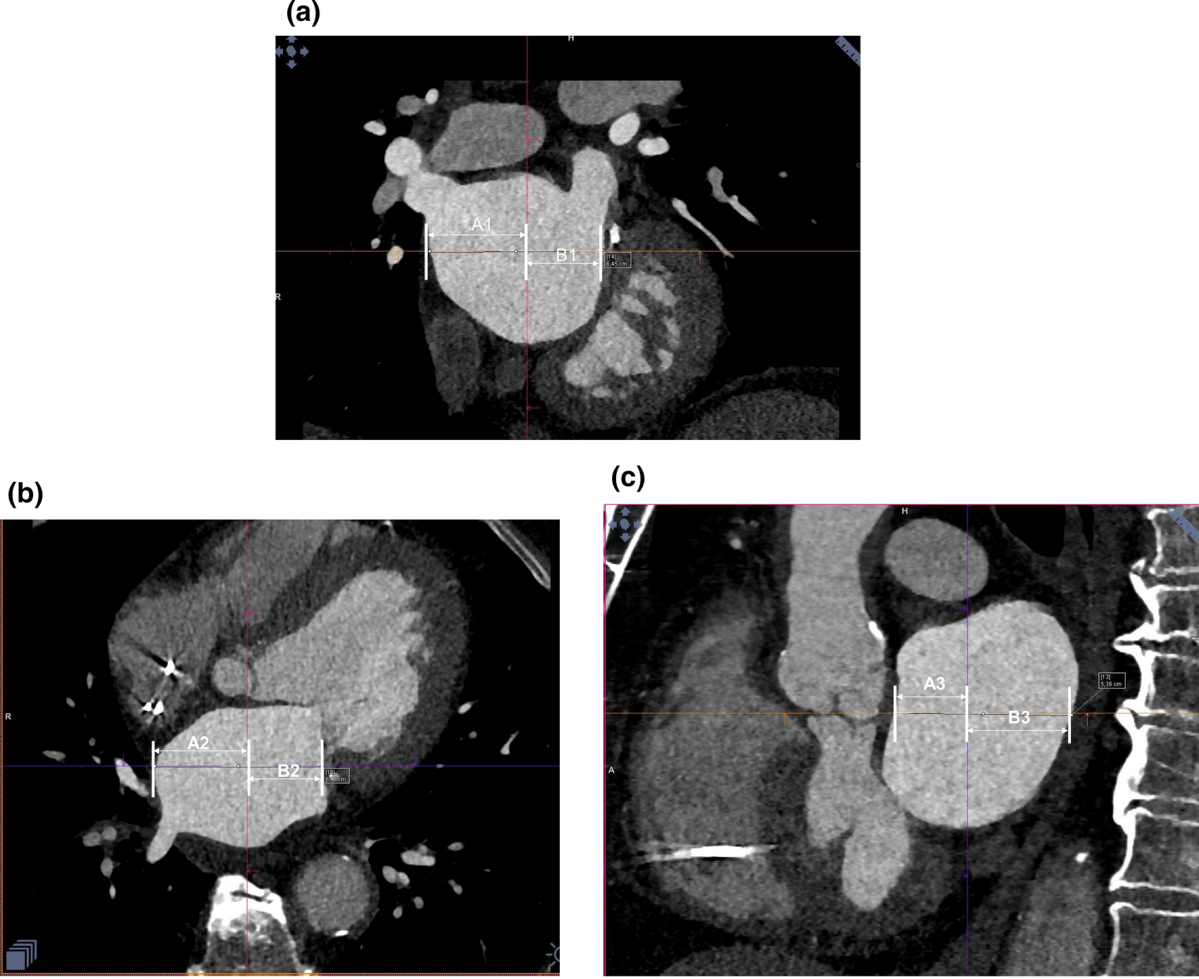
Three planes of left atrium (LA) on computed tomography, based on the principal axis (LA transverse diameter, LA superior-inferior dimension and LA anteroposterior diameter), and the center of mass; A1 + B1 = LA transverse diameter (a); A2 + B2 = LA superior-inferior dimension (b); and A3 + B3 = LA anteroposterior diameter (c) divided by the center of mass of LA.
